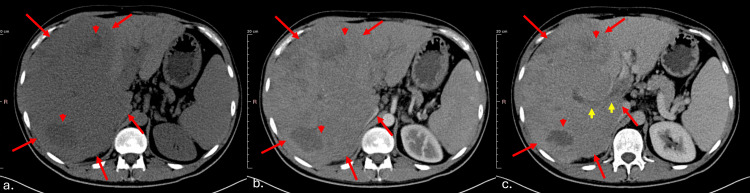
Abdominal CT scan: (a) non-enhancement, (b) arterial phase, and (c) portal phase A voluminous tumoral mass (red arrows) occupying almost the entire right hepatic lobe, with lobulated borders, hypodense appearance on the native phase, inhomogeneous contrast enhancement, and areas of necrosis (red arrowheads). Portal vein thrombosis is also observed (yellow arrows). CT: computed tomography
